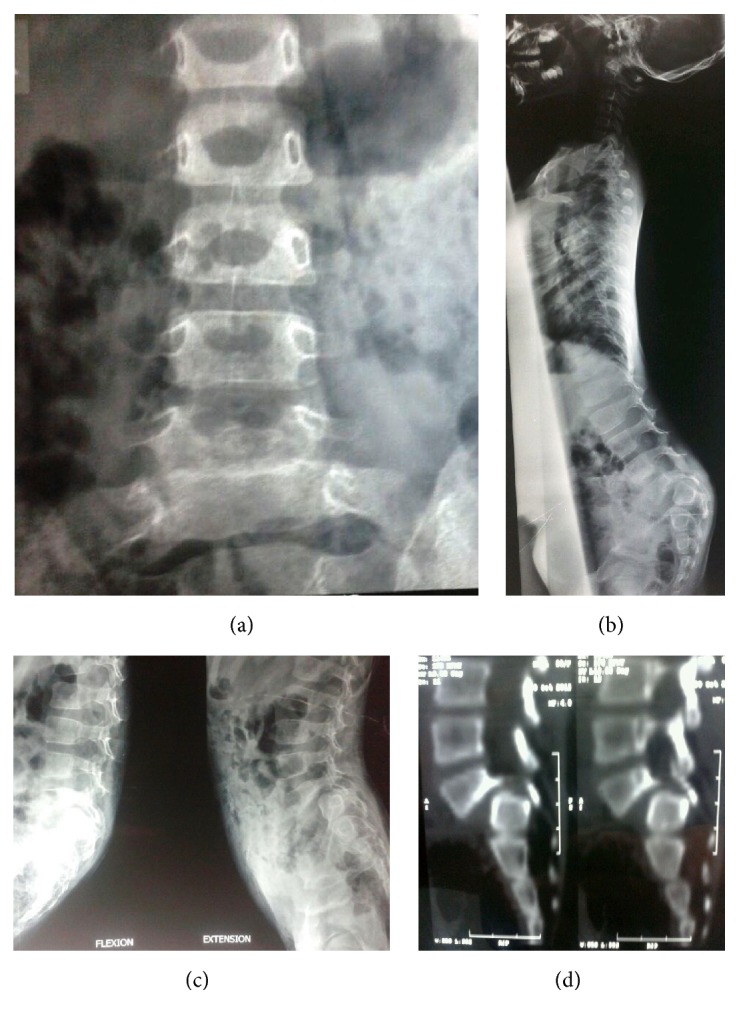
(a) Preoperative A-P radiograph lumbosacral spine. (b) Whole spine standing lateral view radiograph. (c) Flexion-extension lateral view of lumbosacral spine. (d) Sagittal reconstructed CT scan.
